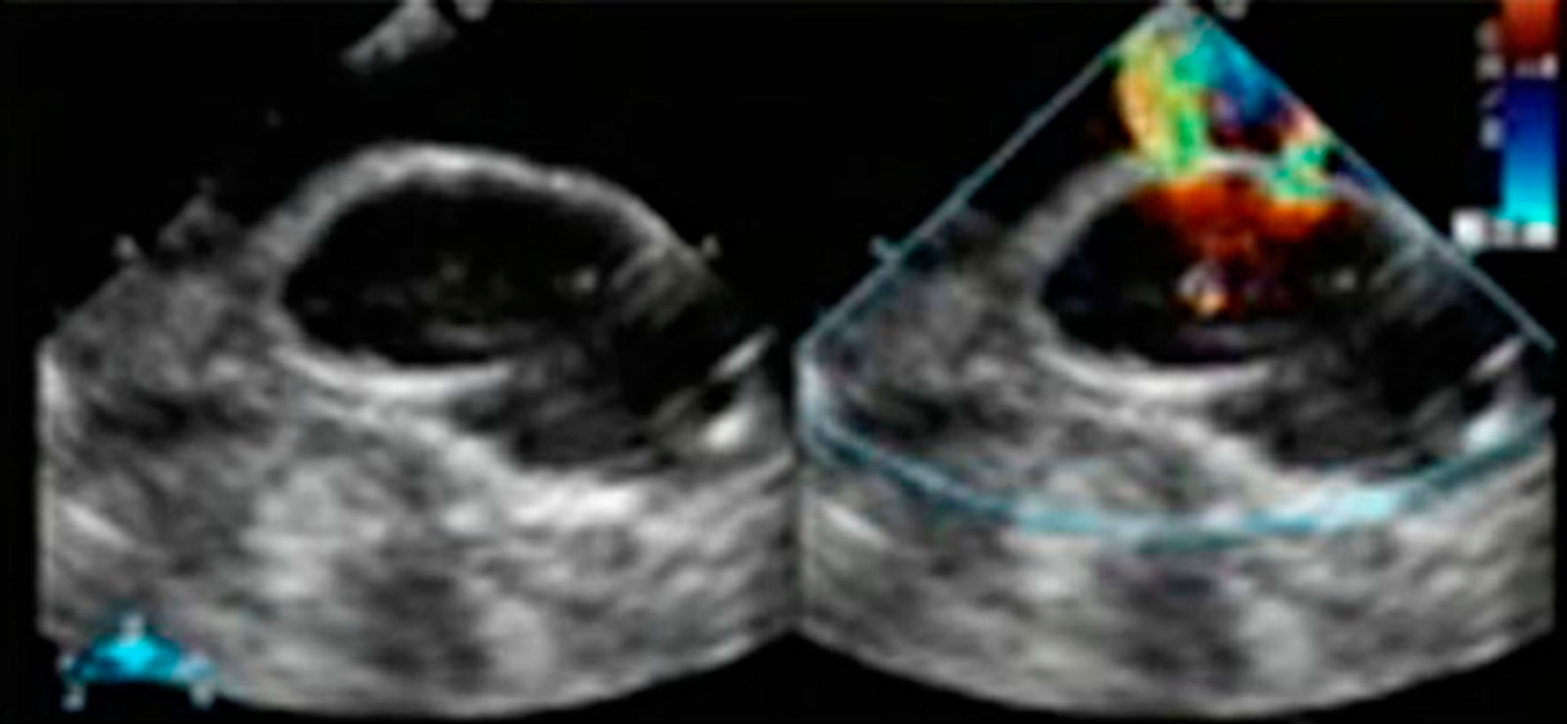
Epi-aortic echocardiography showed shunt flow from the two punch holes of the proximal anastomoses at the CABG
